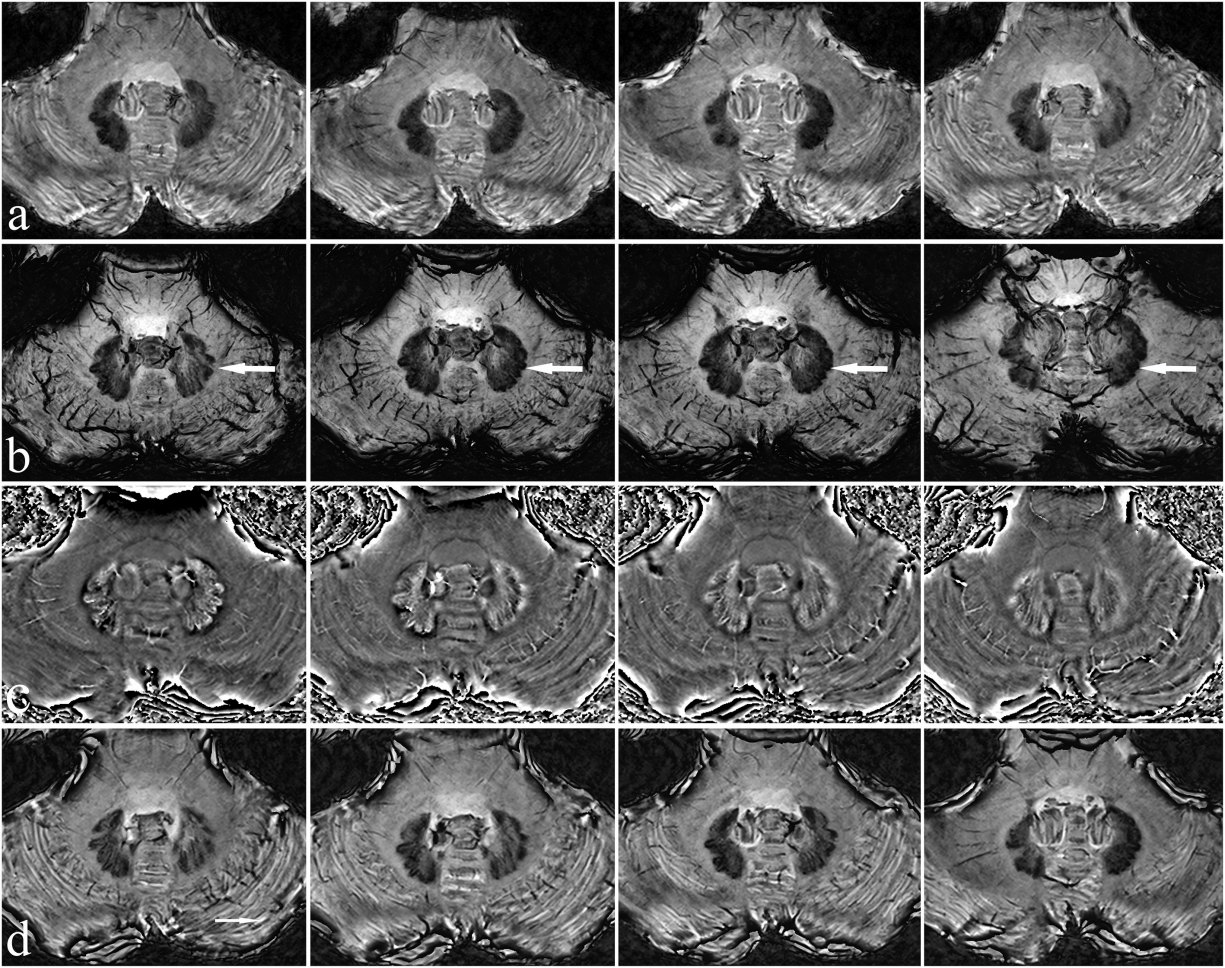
Characteristics of the patient's dentate nucleus on 7T MRI. There is a clear view of the dentate nucleus of the cerebellum. Mag (a), Mip (b), Pha (c), and SWI images (d) have different characteristics, with Mip images being the clearest (see arrow).
